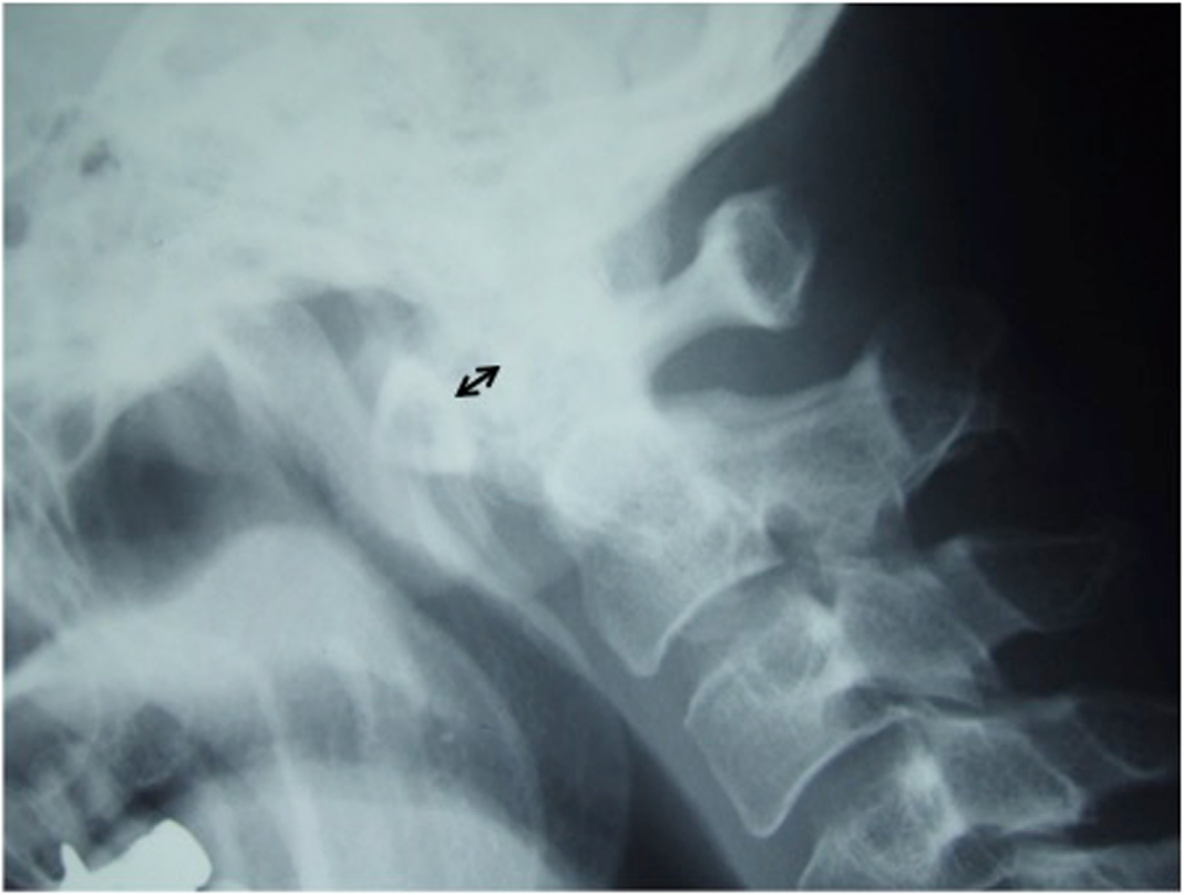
Plain lateral radiograph on admission. The atlantoaxial distance is 7mm (*bidirectional arrow*)
